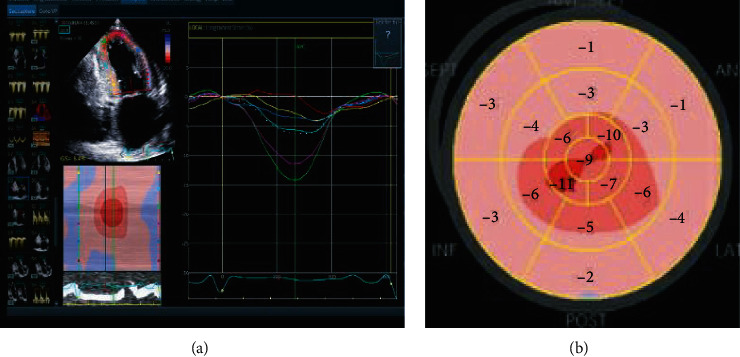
Decreased global longitudinal strain pattern on echocardiogram. There is apical sparing of longitudinal strain, a finding typically seen in cardiac amyloidosis.
